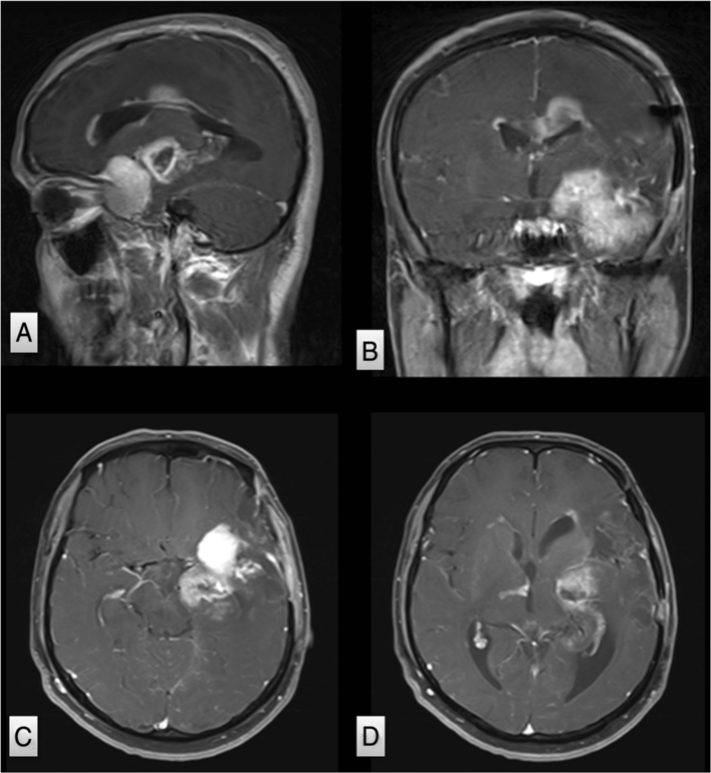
MRI study performed 5 months from the first surgery. Post-contrast parasagittal (a), coronal (b), and axial (c, d) MRI scans were performed demonstrating no change in the size of the meningioma, but there are multiple ring enhancing lesions in the medial temporal lobe adjacent to the meningioma, and in the infarcted tissue involving the corpus callosum
